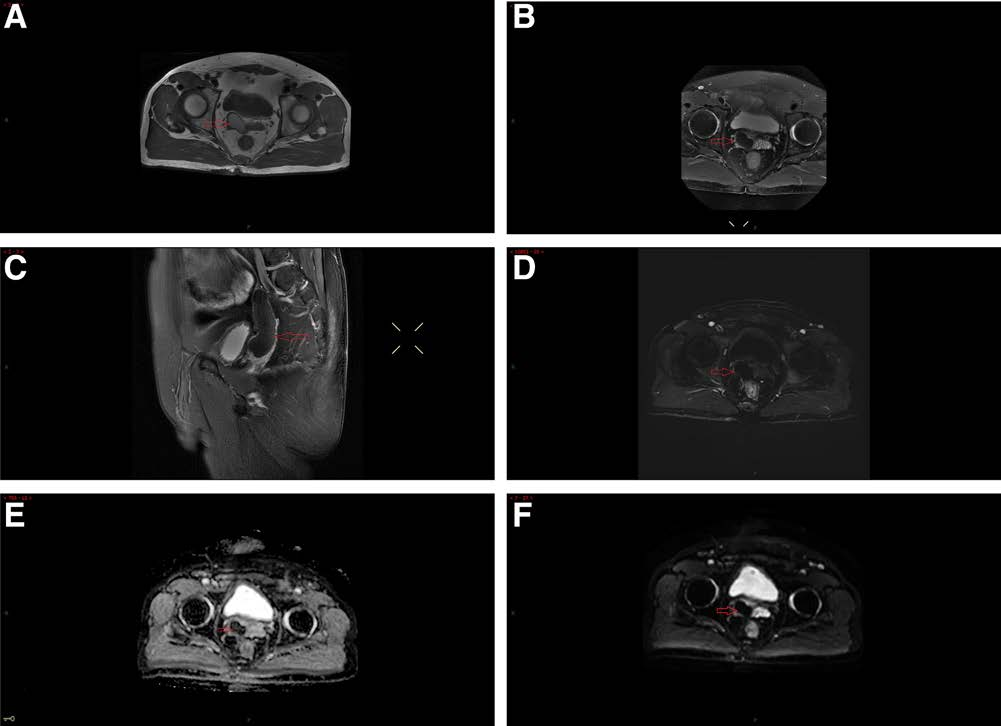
Contrast-enhanced pelvic magnetic resonance imaging (MRI) confirmed a (A) short T1, (B) T2, and (C) signal shadow(arrow), similar to a cord above the right seminal vesicle cyst(arrow). The boundary was clear, with the upper part leading to the “renal region” and the lower part connecting to the right seminal vesicle cyst (arrow). (D) Contrast-enhanced MRI revealed local parenchymal cysts with cyst wall enhancement, but no intrathecal enhancement (arrow). (E) ADC showed low signal intensity (arrow), (F) DWI showed low signal intensity (arrow).
